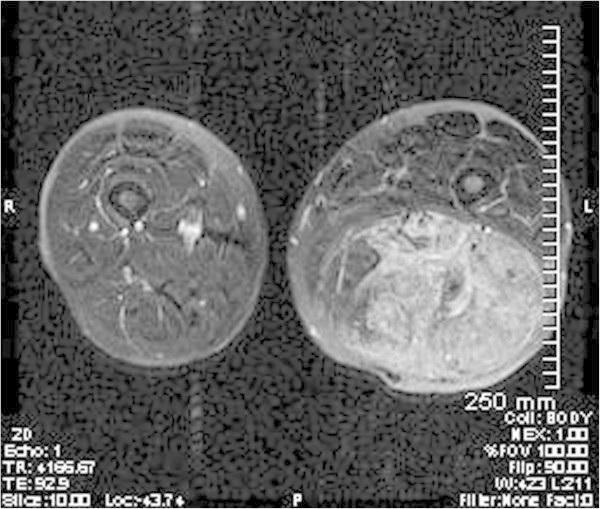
A T2- weighted axial MR image showing avid enhancement of soft tissue components throughout the region indicating presence of various mesenchymal components including fat. Multiple serpiginous enlarged draining and feeding vessels can also be identified.
